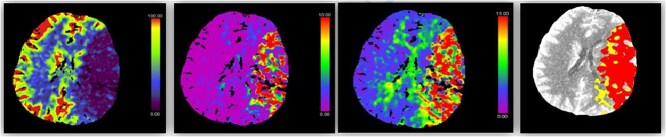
CT brain perfusion study shows a large area of matched perfusion defect in left frontotemporal parietal lobes representing infarct core with surrounding penumbra is noted.
